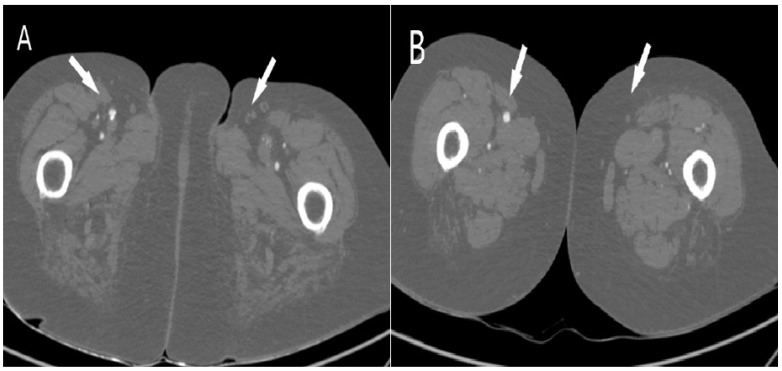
Axial computed tomography angiography shows (A) occlusion of the left common femoral artery (white arrow) and (B) shows occlusion of the left superficial femoral artery (white arrow).
